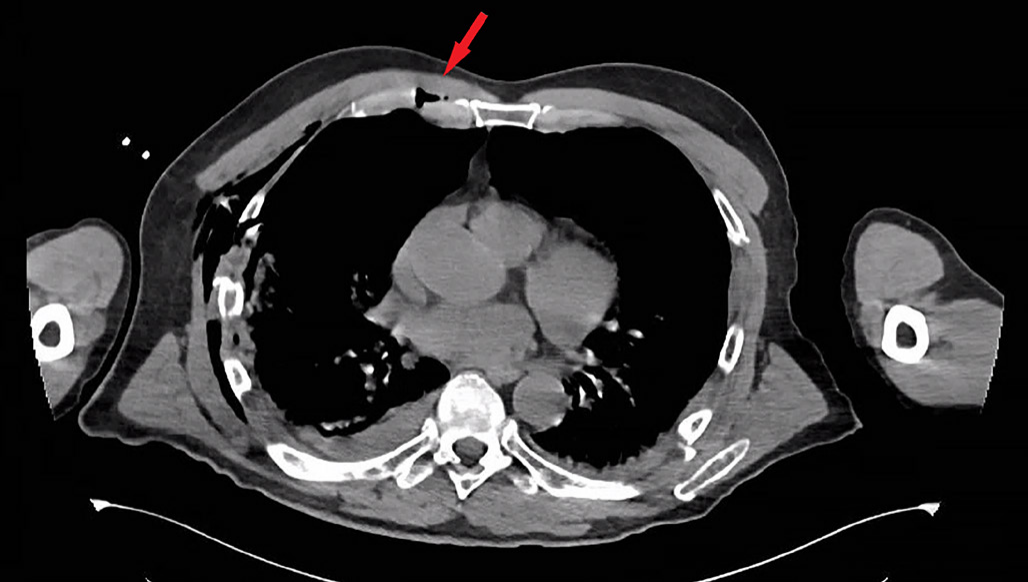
CT scan displaying the costochondral dislocation of the right third rib (arrow).
